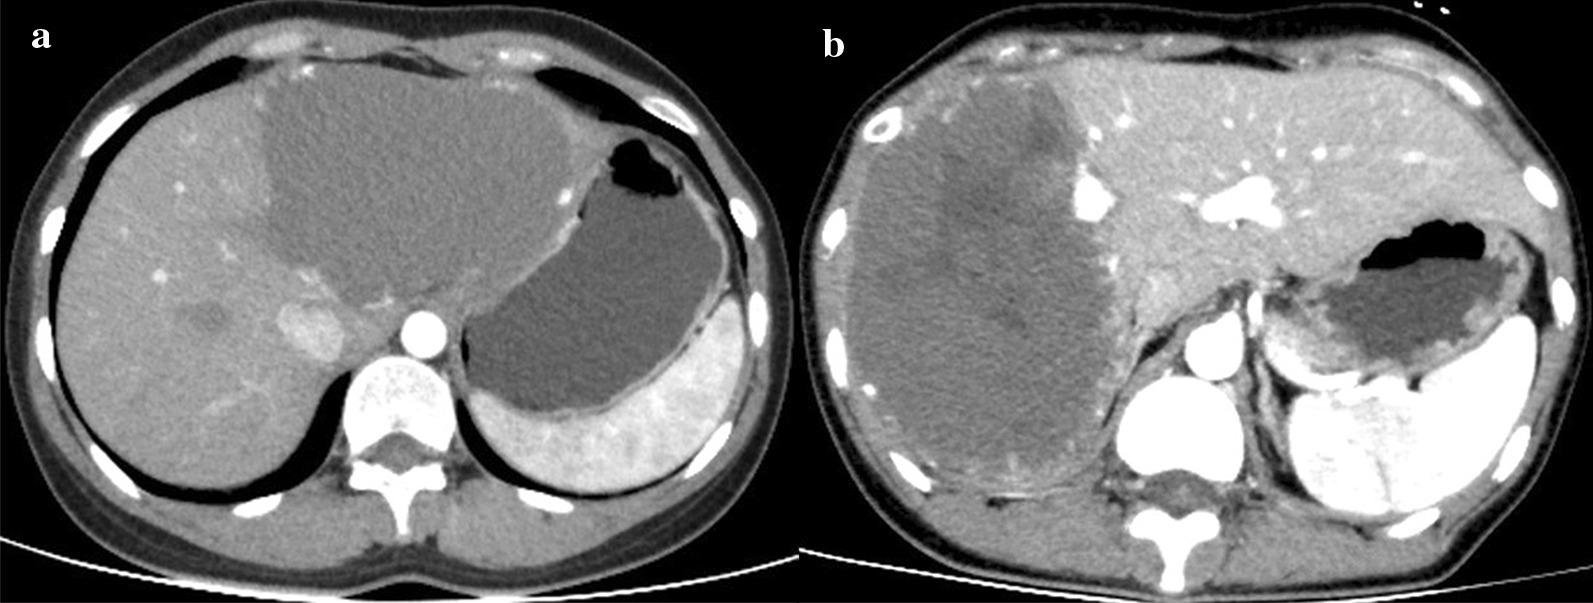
Contrast-enhanced computed tomography of the abdomen: a A peripherally located hemangioma (S2 and S3) excised by EN. b A centrally located hemangioma (S7 and S8) excised by right hemihepatectomy
